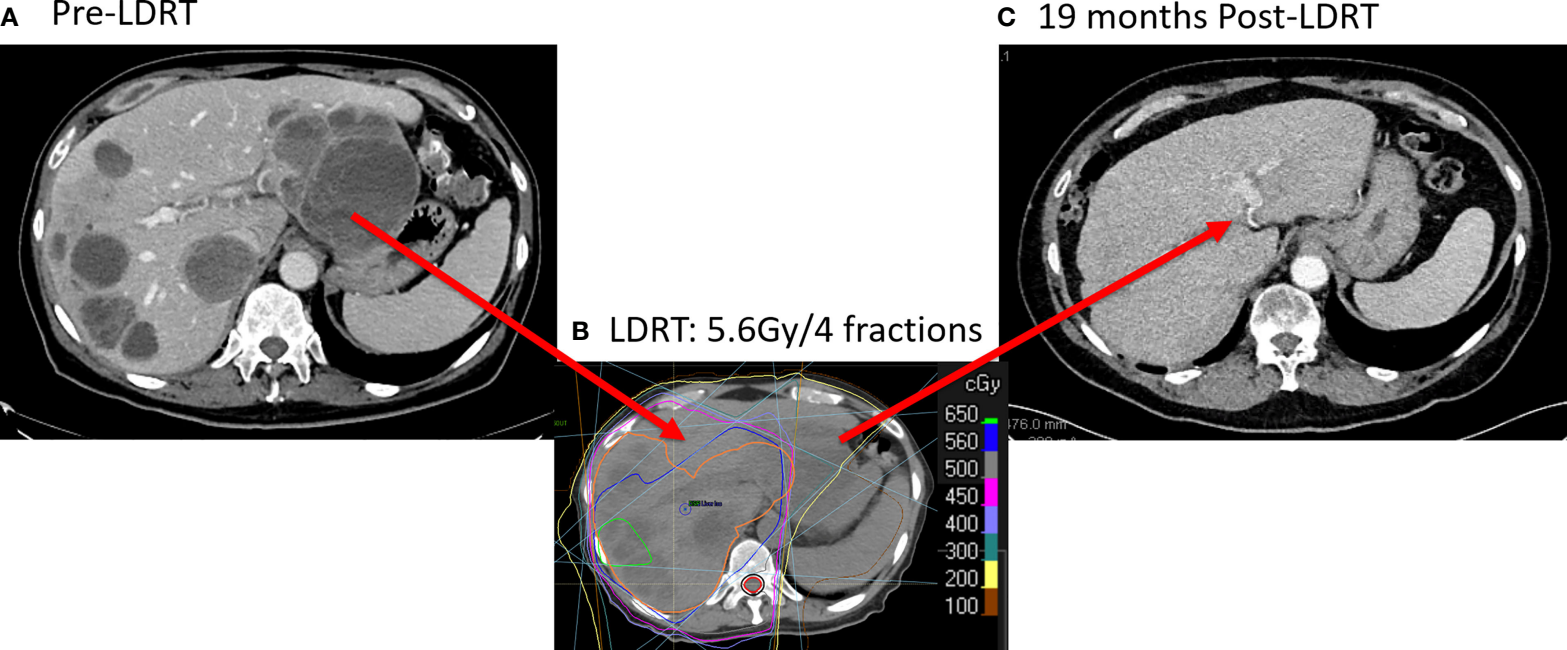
Complete Response with LDRT to Liver metastases. (A) CT scanning (9/4/2019) before LDRT showed multiple liver metastases. (B) The patient received 50 Gy/4 fractions to a lung lesion and 5.6 Gy/4 fractions to nearly the entire liver from 10/8/2019 to 10/11/2019. (C) 19 months after LDRT, CT scans (4/19/2020) showed a complete response in the liver.
